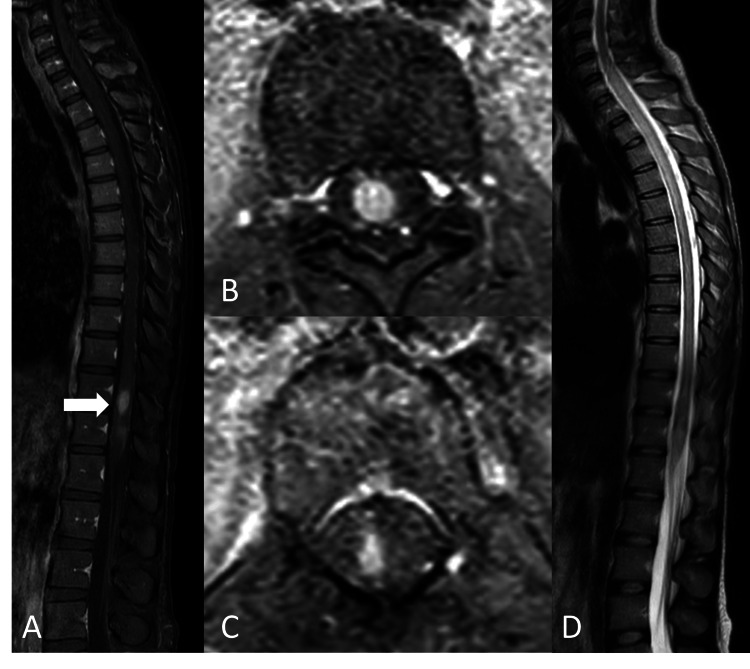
(A, B, C) Whole spine MRI with sagittal and axial planes of T1W fat suppression with gadolinium enhancement sequences demonstrating a few nodular enhancing intramedullary lesions involving the spinal cord at the T11 level to the conus medullaris about the central region (white arrow). (D) Sagittal T2W sequence showing a non-enhancing longitudinal T2 hyperintense signal change intramedullary lesion involving a central portion of the spinal cord extending from C6 to the conus medullaris.
